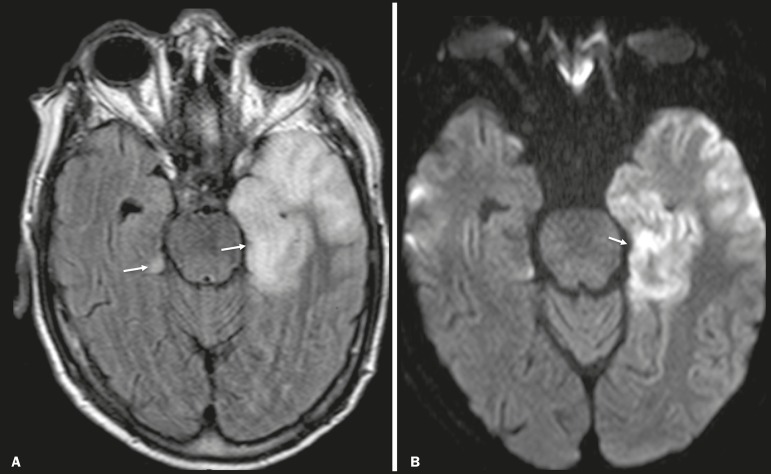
Herpes simplex encephalitis. Axial FLAIR sequence (A) and DWI sequence (B), showing a hyperintense signal that is diffuse in the left temporal lobe and less pronounced in the right temporal lobe, with mesial involvement and restricted diffusion.
